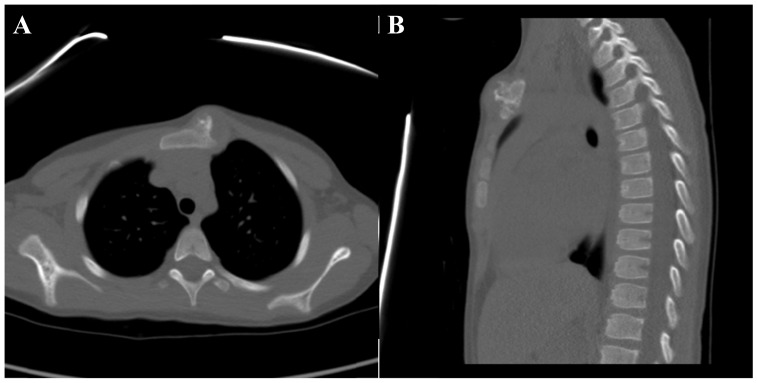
CT scan of the chest at initial presentation, including axial Fig. (2A) and a sagittal Fig. (2B) views.
